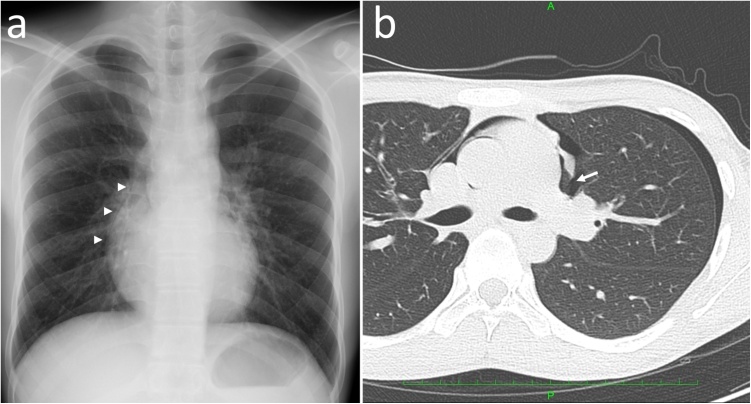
(a) The chest X-ray scan shows left pneumothorax and abnormal air density along the right border of the cardiac shadow (arrow heads). (b) The chest computed tomography scan reveals left pneumothorax, pneumopericardium, and a partial defect of the left pericardium (arrow).
